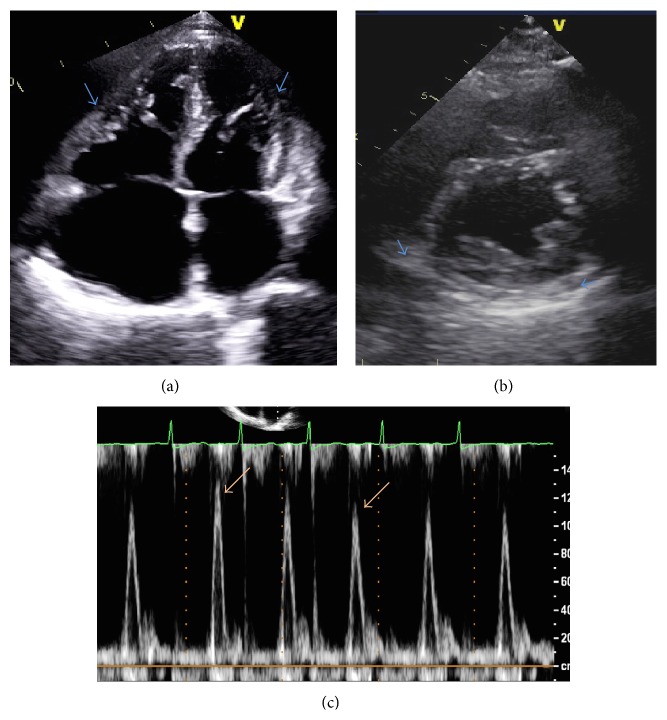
(a), (b) Transthoracic echocardiogram (apical (a) and parasternal short-axis (b)) showing resolution of pericardial effusion (blue arrows) after 10 weeks of IV Penicillin. (c) Pulsed-wave Doppler interrogation of mitral valve inflow on transthoracic echocardiogram: normal variation of mitral valve E velocity (orange arrows), suggesting normalization of pericardial pressure.
